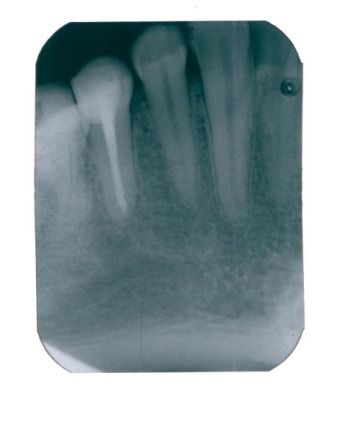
Follow-up radiograph, one year after root canal therapy, the resolution of the periradicular bone lesions is evident
